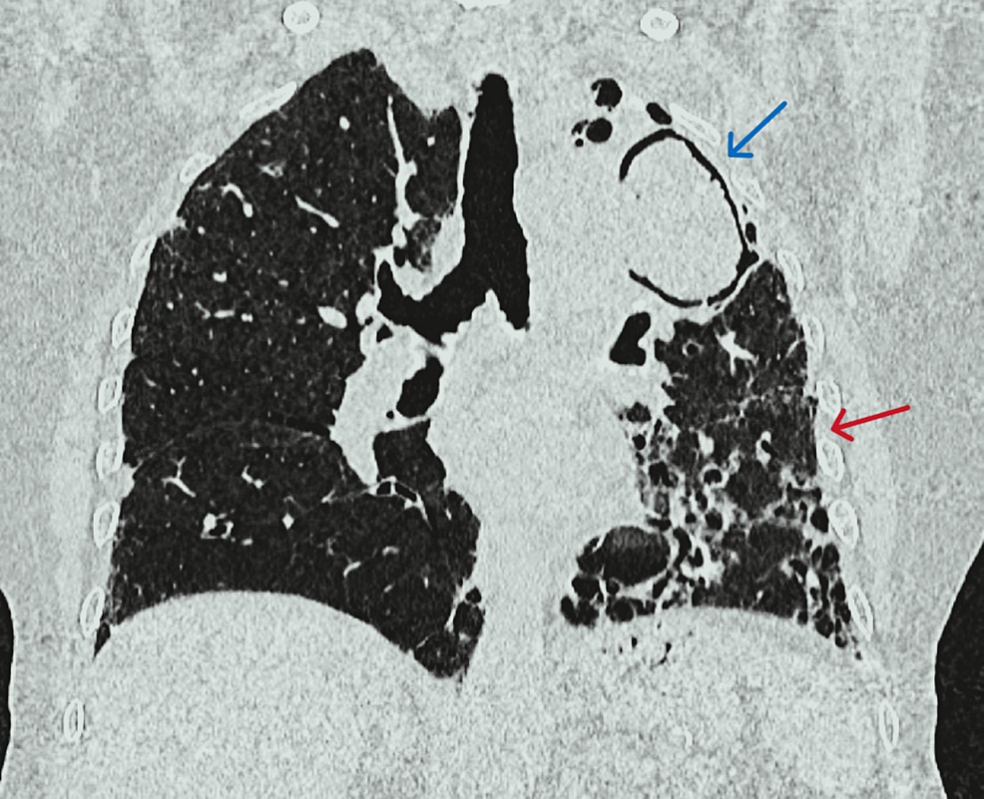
High-resolution computed tomography (HRCT) thorax showed a large cavity with an intracavitary fungal ball (blue arrow) in apicoposterior segment of the left upper lobe (67×40×60 mm) with soft tissue density area within and adjacent areas of consolidation, fibrosis and traction bronchiectasis (red arrow)in the left upper lobe.
